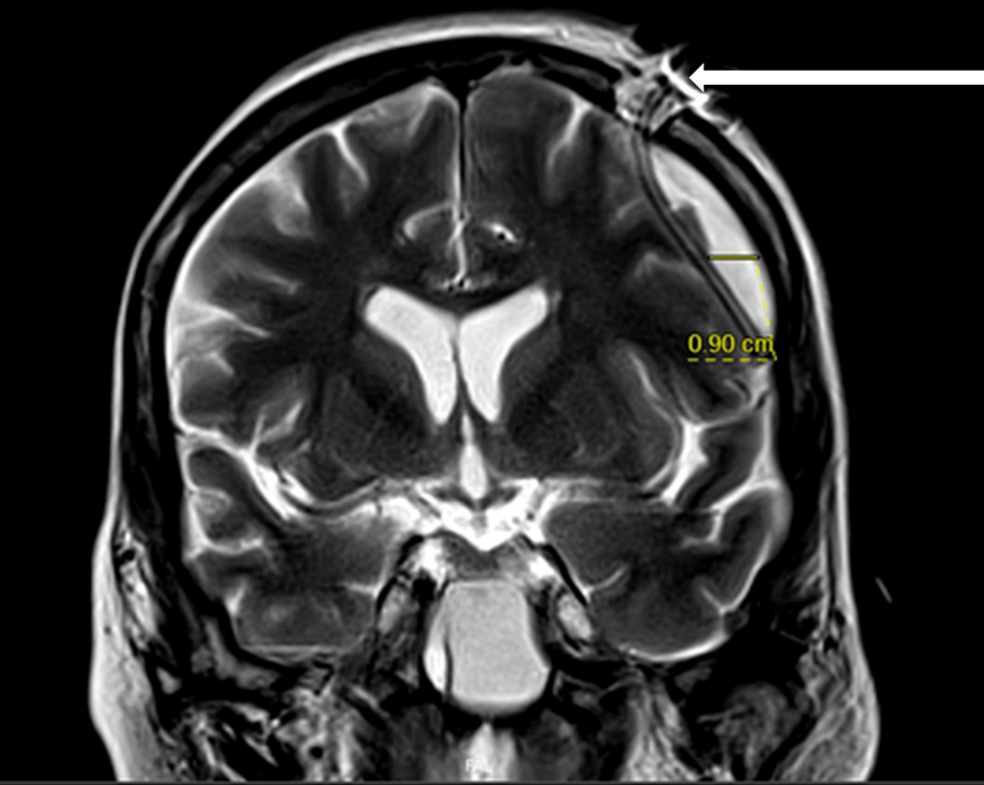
Magnetic resonance imaging status post burr hole procedure with placement of a catheter drain (white arrow).
